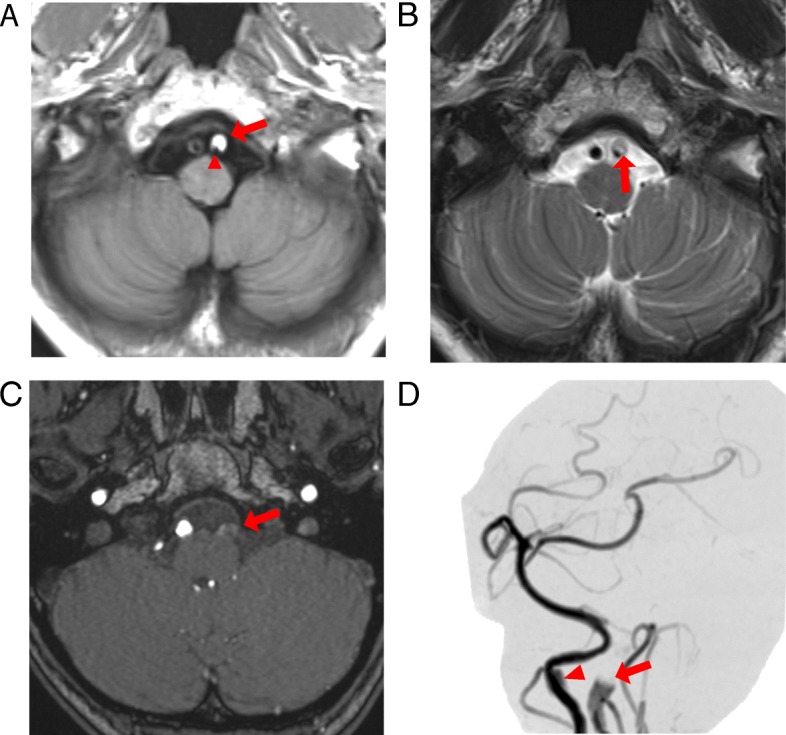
3T magnetic resonance imaging of the head. a T1-weighted images showed a high-signal intensity lesion (arrow) and narrowing of the residual signal void (arrowhead) of the left vertebral artery. b T2-weighted images showed a curvilinear high-signal structure and an intimal flap (arrow), suggesting left vertebral artery dissection and intramural hematoma. c and d Three-dimensional time-of-flight magnetic resonance angiography showed obstruction of the left vertebral artery (arrow), an aneurysm related to dissection of the right vertebral artery (arrowhead)
